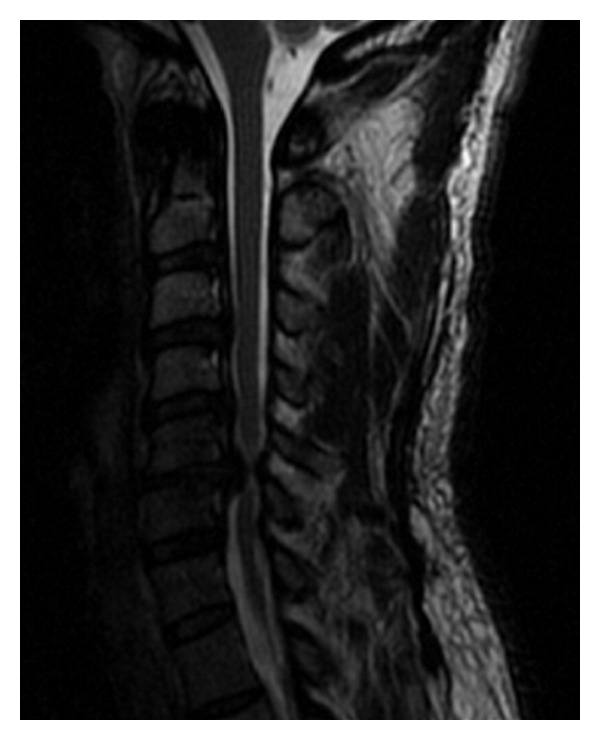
Preoperative MRI sagittal showing large area of high signal intensity centered behind the massive C5-6 herniated disc.
